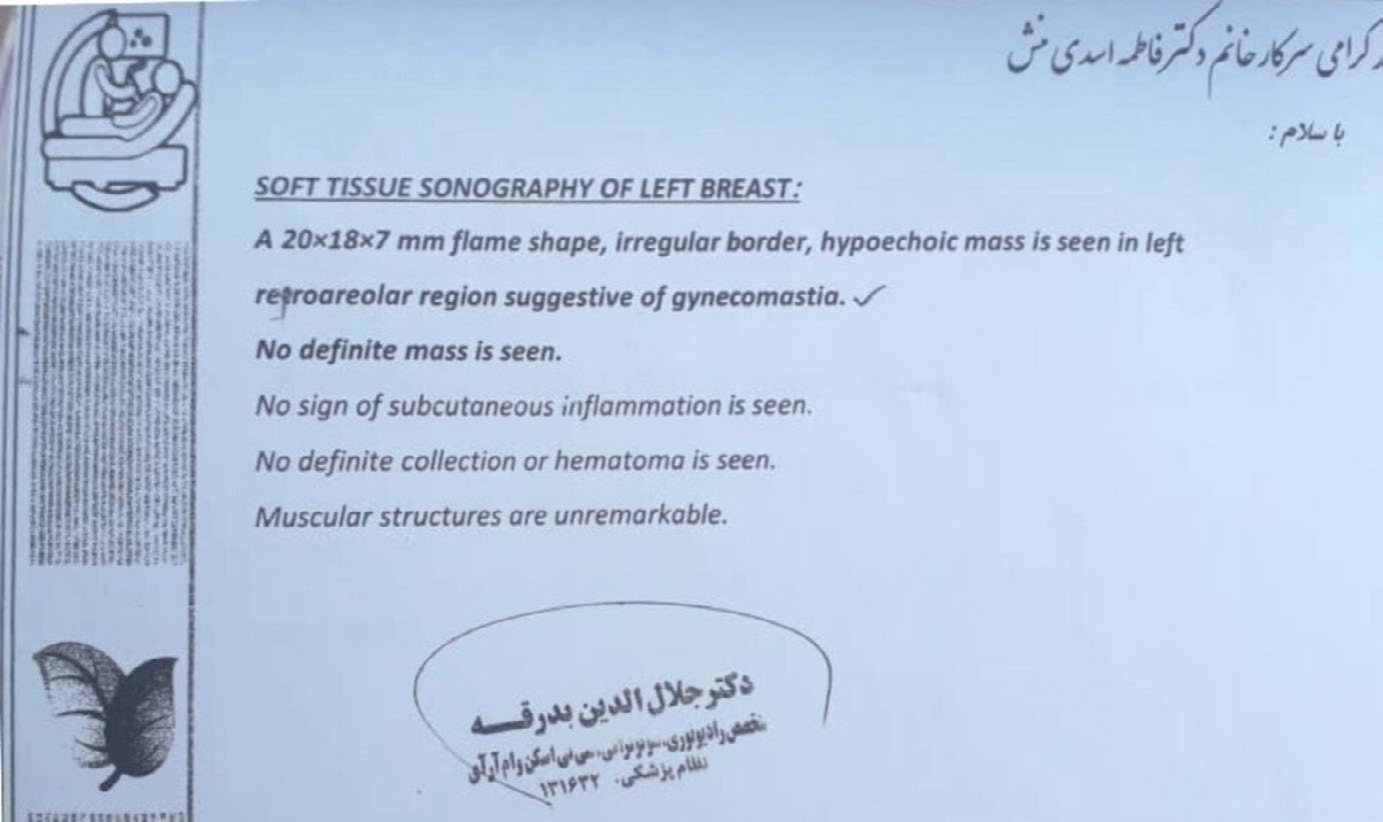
Sonography report of the patient's left breast. The characteristics of the breast mass are shown in the figure.
